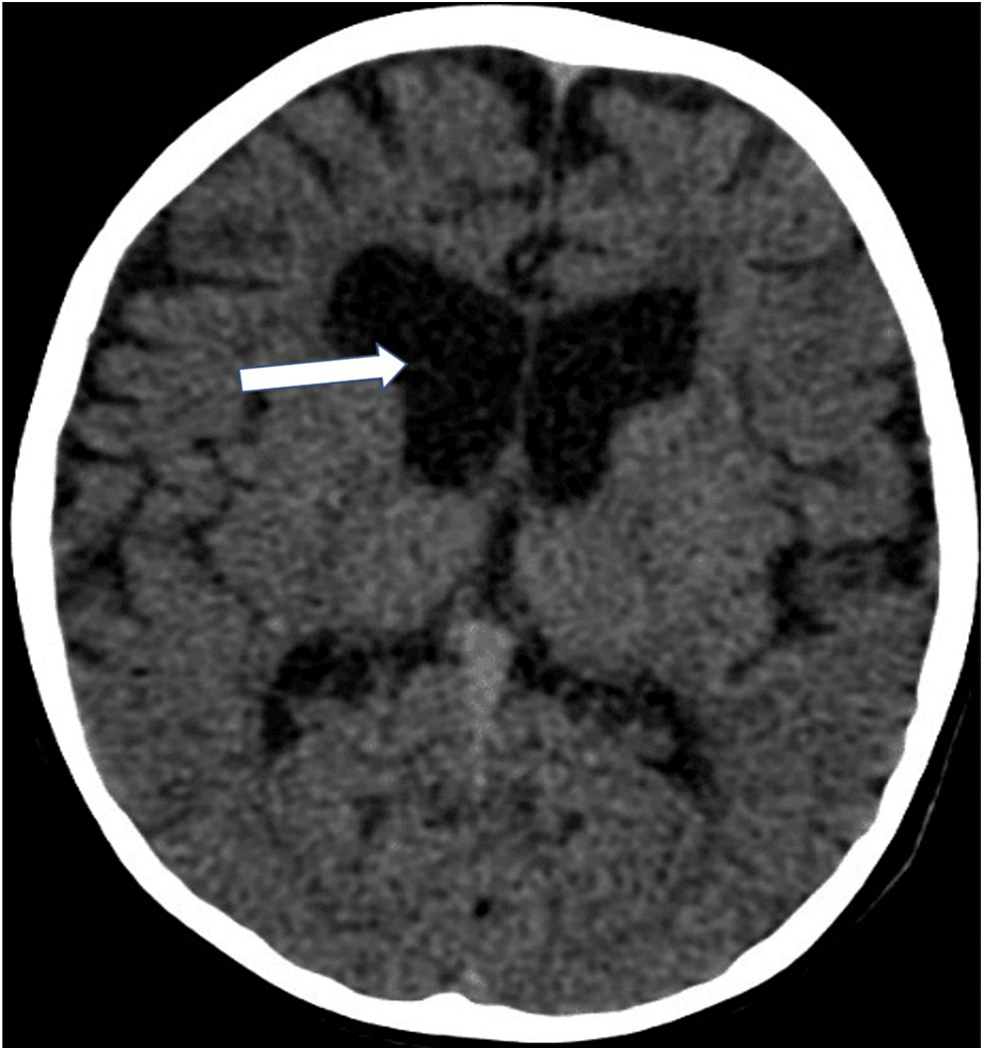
Non-enhanced CT brain axial section demonstrates dilated bilateral lateral ventricles CT: computed tomography
